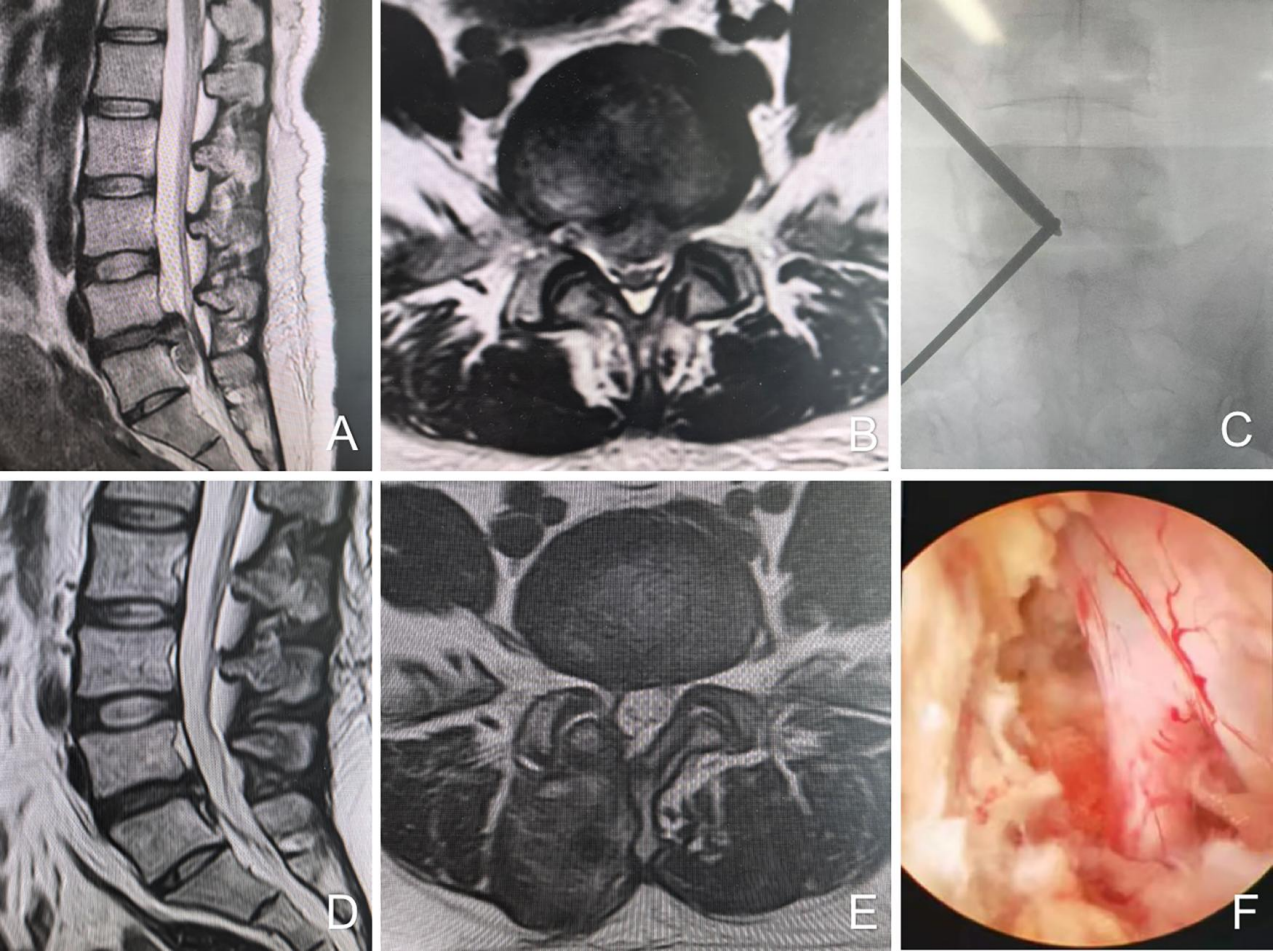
Female, 39 years old, L5/S1 lumbar disc herniation. (A) Preoperative sagittal MR image showed L5/S1 lumbar disc herniation; (B) preoperative axial MR image showed herniated lumbar disc compressed nerve root and dural sac; (C) intraoperative fluoroscopic confirmation of metal rods; (D) postoperative sagittal MR image shows complete decompression of the spinal canal, but with intraspinal adhesions; (E) postoperative axial MR image showed the complete removal of herniated disc and bony fragment; (F) intraoperative image after complete neural decompression.
